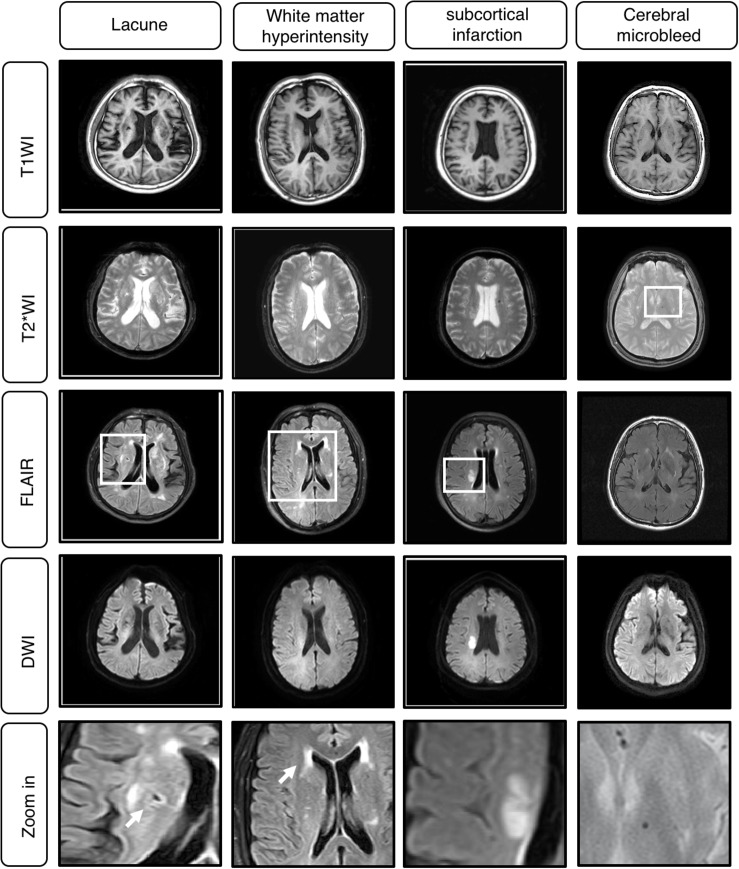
Example cases of Cerebral Small Vessel Disease (CSVD) MRI A. Classical MRI of CSVD including lacune, white matter hyperintensity (WMH), subcortical infarction, cerebral microbleed.
